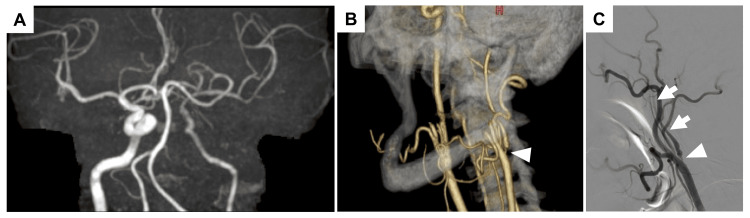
Imaging findings of near occlusion in the left ICA A: Head MRA showing poor visualization of the left ICA; B: Neck CTA demonstrating near occlusion of the left ICA. The arrowheads indicate the point of maximal stenosis; C: Left CCA angiogram showing near-occlusion and collapse of the left ICA. The arrowhead marks the point of maximal stenosis, and the arrows indicate a collapsed segment of the left ICA. ICA: internal carotid artery; CCA: common carotid artery; CTA: computed tomography angiography; MRA: magnetic resonance angiography
